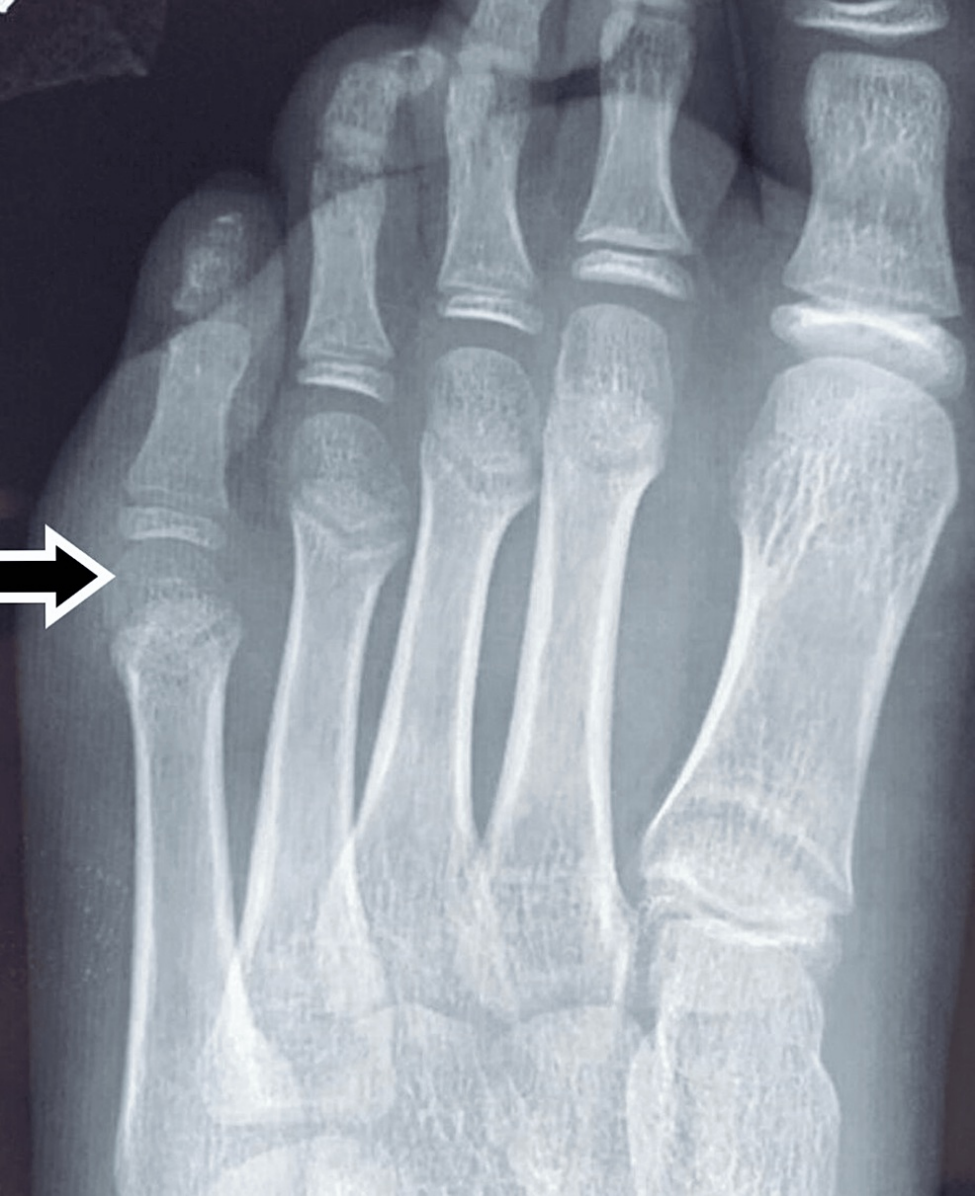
X-ray after two years of follow-up with maintained reduction
